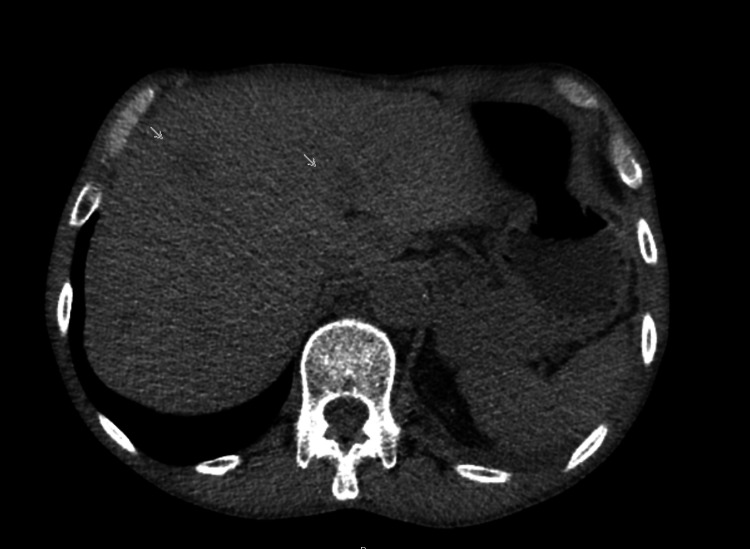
Liver metastasis During the staging of the carcinoma, multiple liver metastases were apparent in the abdominal CT scan; two of the largest ones are marked with gray arrows.
